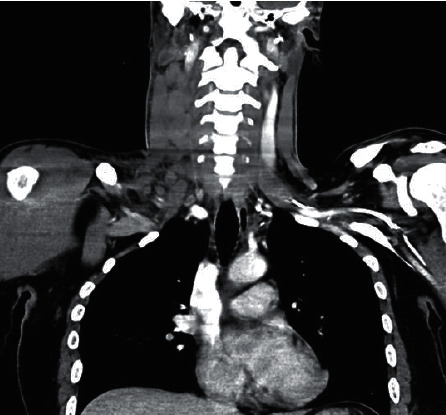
Coronal CT imaging of the neck showing right-sided cervical lymphadenopathy.
